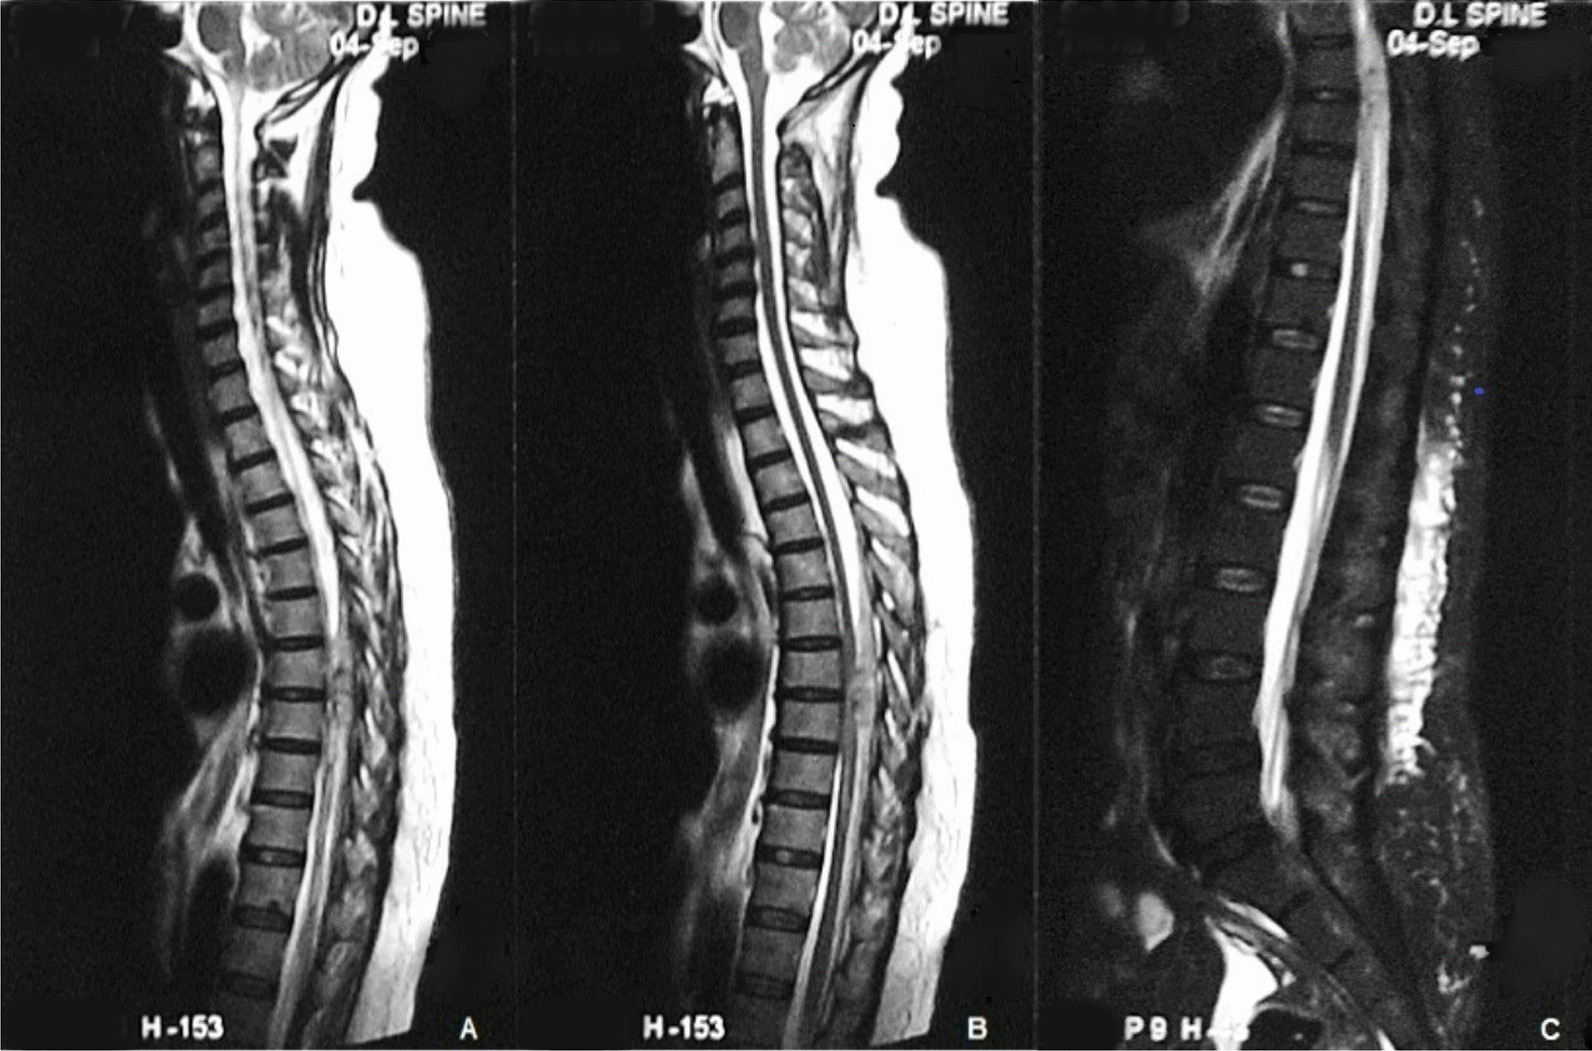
T2-weighted (A and B) and fat-suppressed T2-weighted (C) sagittal images showing intradural extramedullary hematoma at D7–D8 vertebral level
